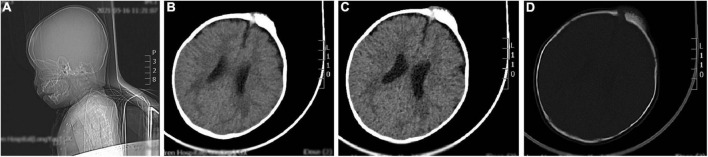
A representative image of the head CT scan shows the irregular bone structure with mixed density mass on the left side of the cranial plate in the median frontal area, slightly reduced density in the bilateral parietal lobes, widening of the ventricles and extracerebral space, and thickening of the septal sinus mucosa. (A) Sagittal view shows a mass in the frontal area. (B,C) Soft tissue window at the central level of the lateral ventricle show a high-density mass in the left frontal region. (D) Bone window at the central level of the lateral ventricle: the bony structure of the left cranial plate in the middle frontal region is irregular, and there is a mixed density mass shadow.
